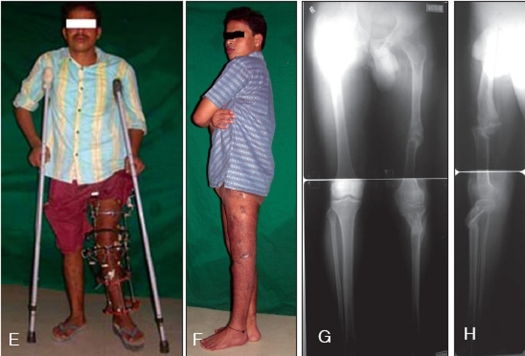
E) The taylor spatial frame fixator permits walking and mobilization when due care is taken. F) Clinical result showing full correction of femoral procurvatum and tibial recurvatum. G) Full length AP X-ray showing complete correction of deformity and mechanical axis passing through the center of the knee. H) Full length lateral X-ray showing the mechanical axis passing through the center of the knee joint even though there is a mild translation of the distal femur anteriorly and distal tibia posteriorly. See text for explanation
